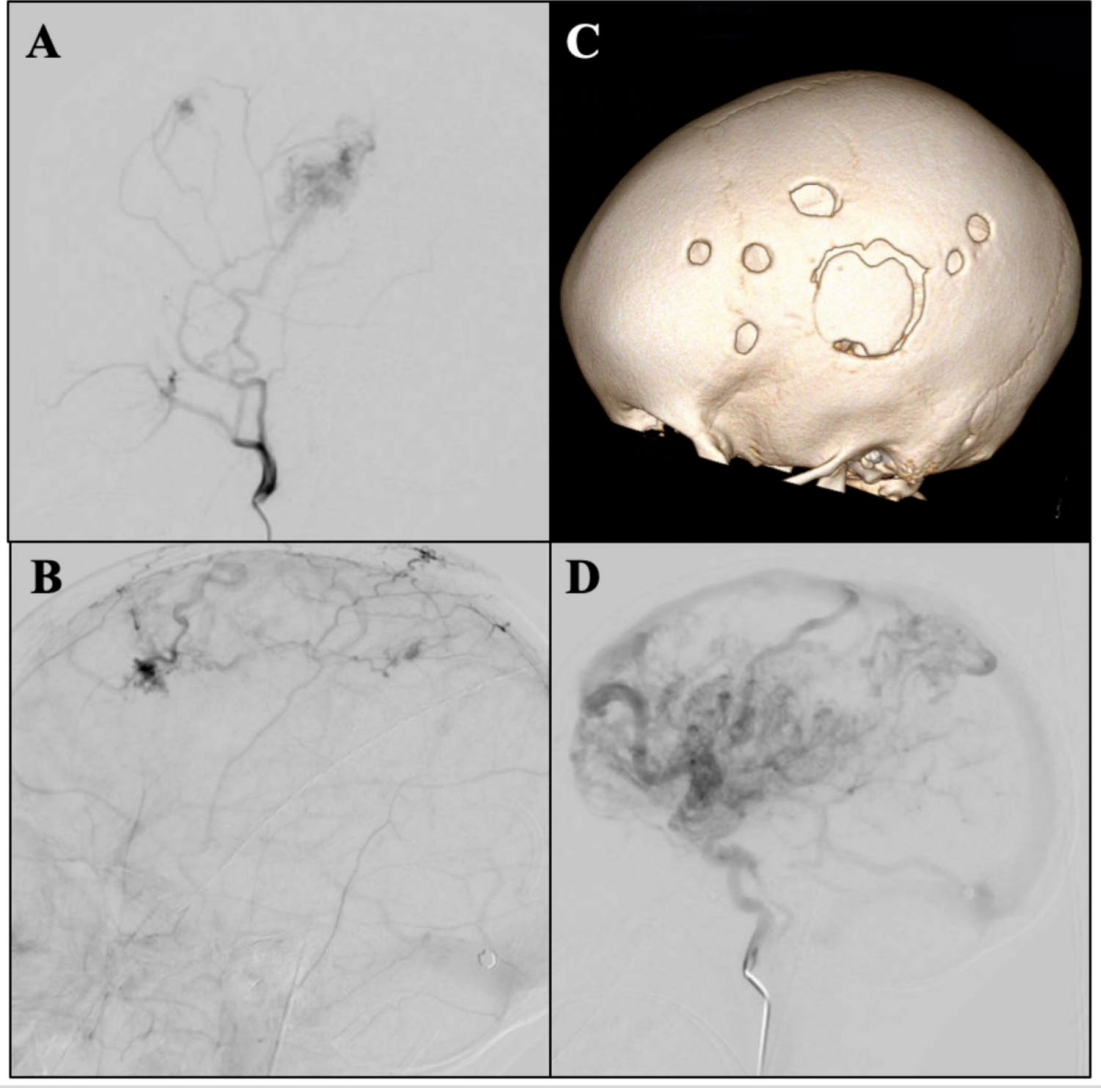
A Lateral right ICA and B lateral right ECA angiogram projections showing proliferation of small vessels through the burr holes as well as a successful EDAS over approximately the right motor cortex compared to the three-dimensional model (C) based on a post-operative CT scan. D Lateral right ICA injection showing innumerable vessels with early venous drainage into hypertrophied veins
